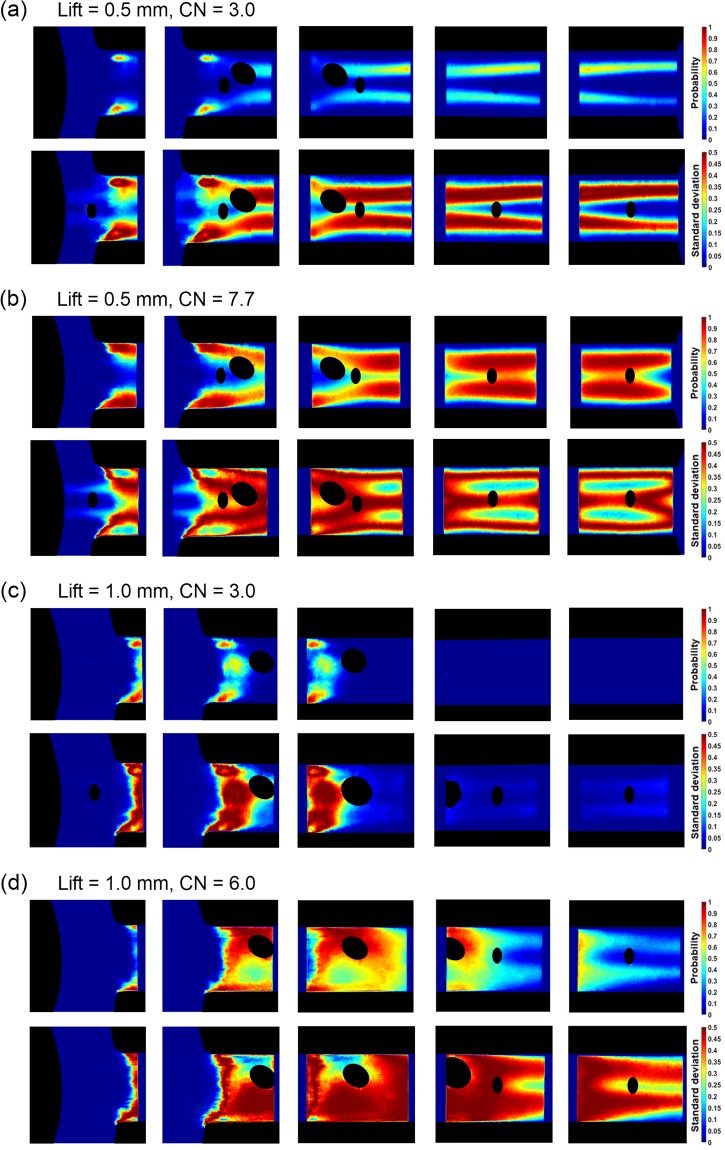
Mean and standard deviation images (top view) for the base blend produced by 16000 radiographs for each irradiated location (Re = 35500). Mean (top frame) and standard deviation (bottom frame) images for (a) lift = 0.5 mm-CN = 3.0, (b) lift = 0.5 mm-CN = 7.7, (c) lift = 1.0 mm-CN = 3.0 and (d) lift = 1.0 mm-CN = 6.0 (maximum CN achievable for the specific lift). The probability values of the legend refer to the vapour presence at each pixel of the plot, while the standard-deviation values denote the probability variation and are indicative of transient cavitating structures. The respective images for the additised blend exhibit similar trends and the same overall qualitative patterns regarding both mean probability and standard deviation. Artefacts attributed to imperfections of the scintillator have been omitted in black colour. Flow is from left to right.
